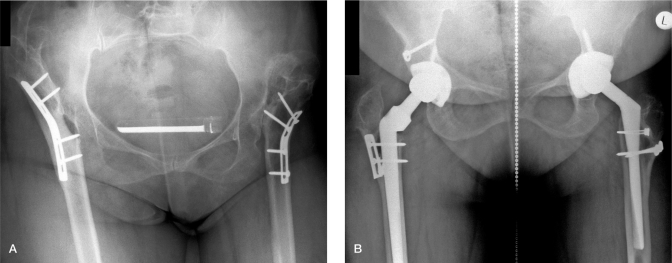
A 54-year-old woman who had high congenital dislocation of both hips. At the age of 17, she had undergone a bilateral high-seated Schanz osteotomy to reduce limp. A. Preoperatively. B. 9 years after a cementless total hip arthroplasty of the left hip and 8 years after a similar procedure on the left hip. Both hips underwent femoral shortening and advancement of the greater trochanter. The leg-length discrepancy was 1 cm (the left side being shorter). There were no radiographic signs of loosening of the components and no signs of polyethylene wear.
